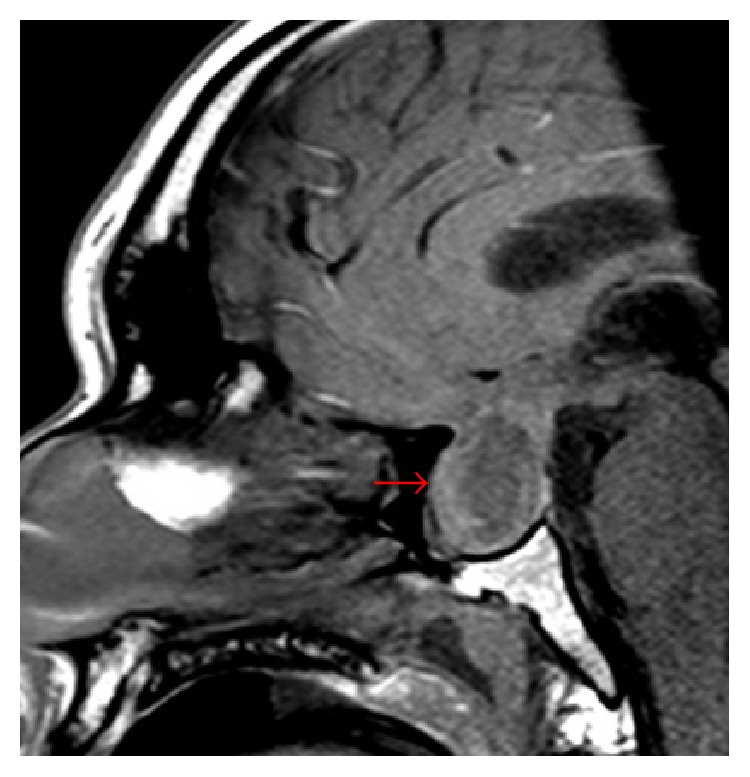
T1 SAG SELLA (MRI brain with and without contrast). Red arrow indicating large pituitary mass.
